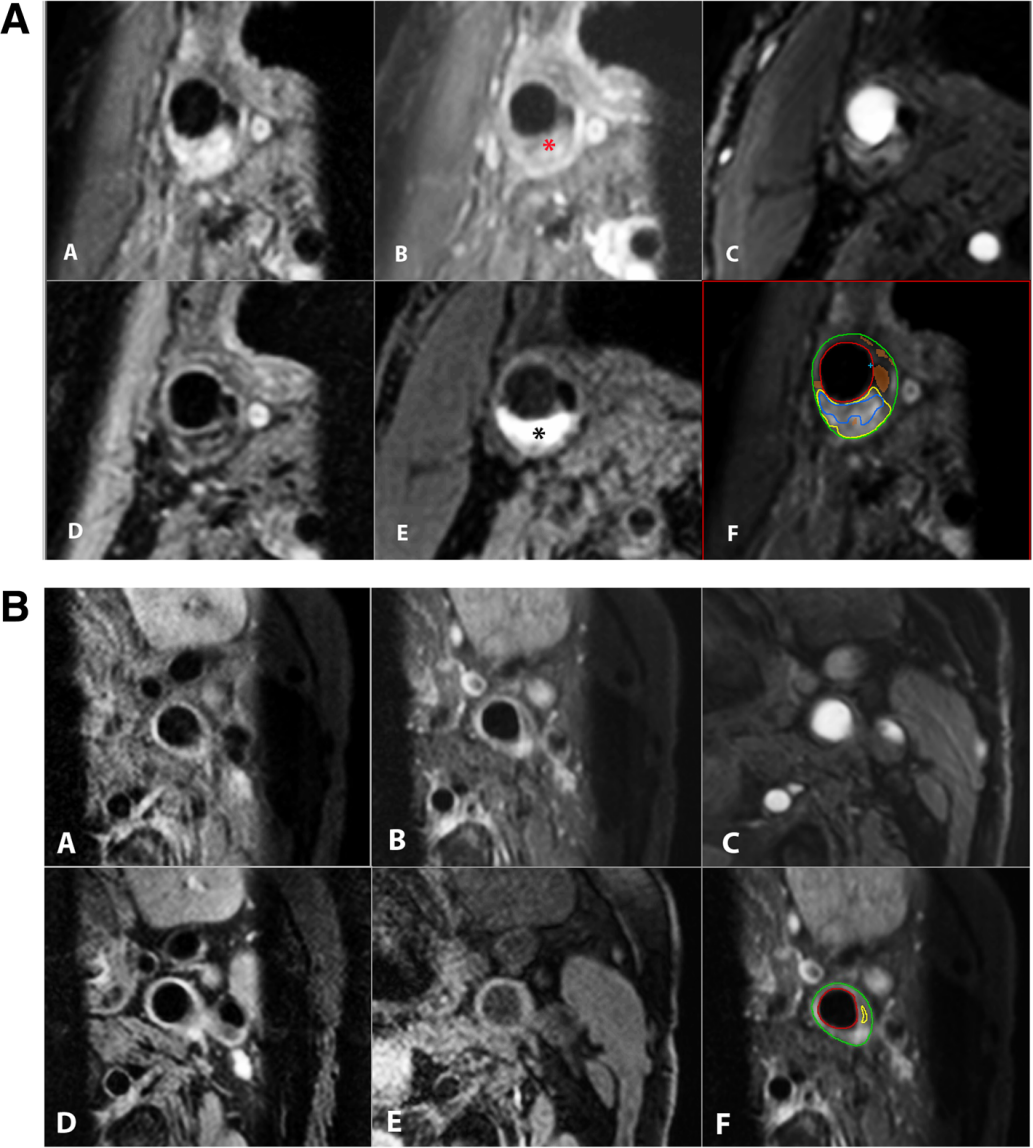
a Transversal cardiovascular magnetic resonance (CMR) images of a patient with carotid plaque in the right carotid artery with intraplaque hemorrhage (IPH). The following CMR sequences were acquired: (A) pre-contrast T1-weighted (T1W) quadruple inversion recovery (QIR) turbo spin echo (TSE), (B) post-contrast T1W QIR TSE, (C) time of flight (TOF), (D) T2W TSE and (E) T1W inversion recovery (IR) turbo field echo (TFE). Three regional saturation slabs were positioned on T1W QIR TSE and T2W TSE sequences; one on the throat to reduce swallowing artefacts and another two on the subcutaneous fat, with an angle of approximately 45 degrees with respect to the regional saturation slab that is positioned on the throat (both left and right) to reduce ghosting. A lipid-rich necrotic core was identified as a region within the bulk of the plaque that does not show contrast enhancement (* on B) on the post-contrast T1W QIR images. On the T1W IR TFE image, a hyper-intense signal in the bulk of the plaque can be clearly observed, indicating the presence of IPH (* on panel E). Panel F shows the plaque contours on the post-contrast T1W QIR TSE images (green = outer vessel wall, red = inner vessel wall, yellow = lipid-rich necrotic core, blue = IPH, orange/brown = calcifications). b Transversal CMR image of a carotid plaque in the right carotid artery without IPH but with a small lipid-rich necrotic core. All panels consist of the same sequences as in Fig. 1a
